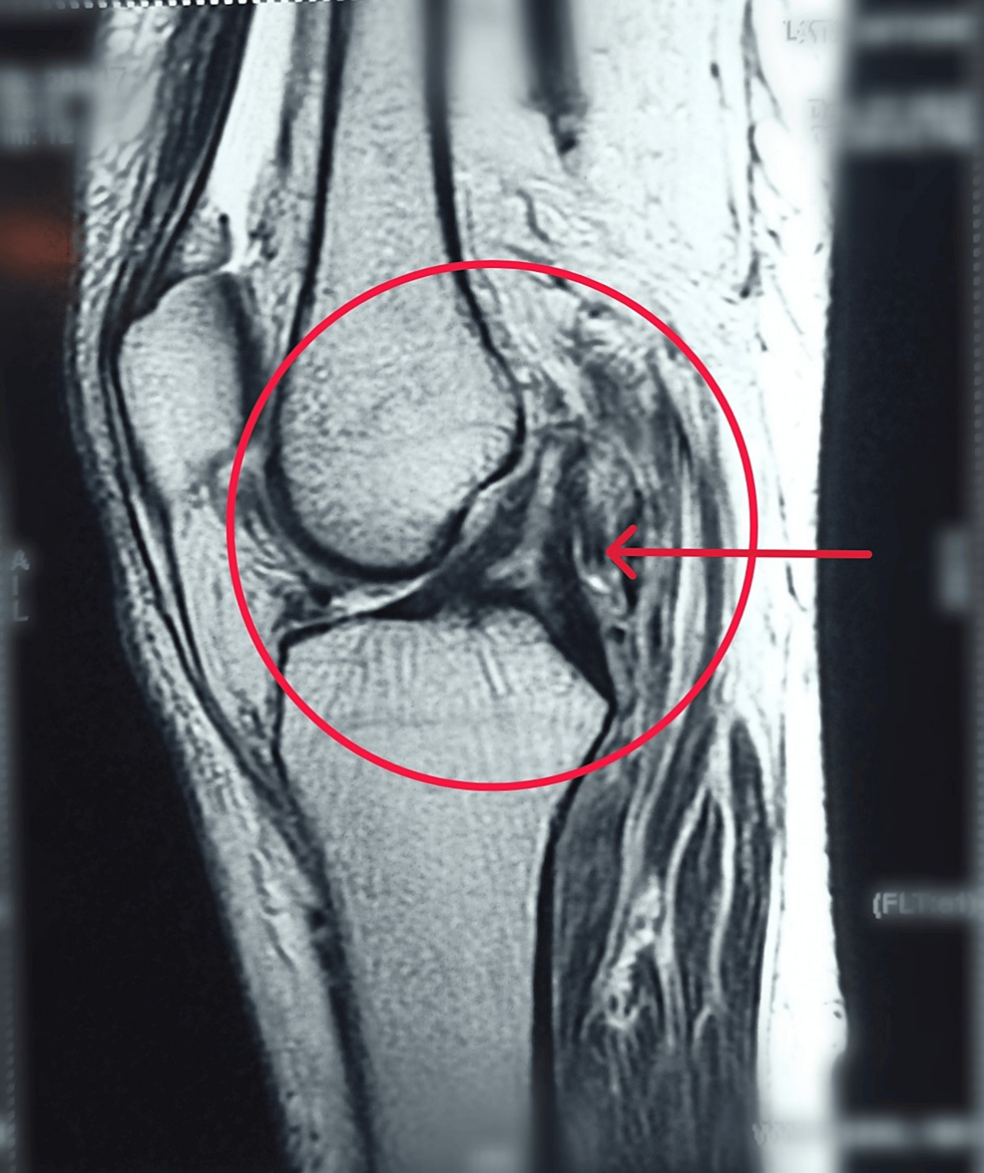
MRI showing ACL and PCL injury MRI: Magnetic resonance imaging; ACL: anterior cruciate ligament; PCL: posterior cruciate ligament
